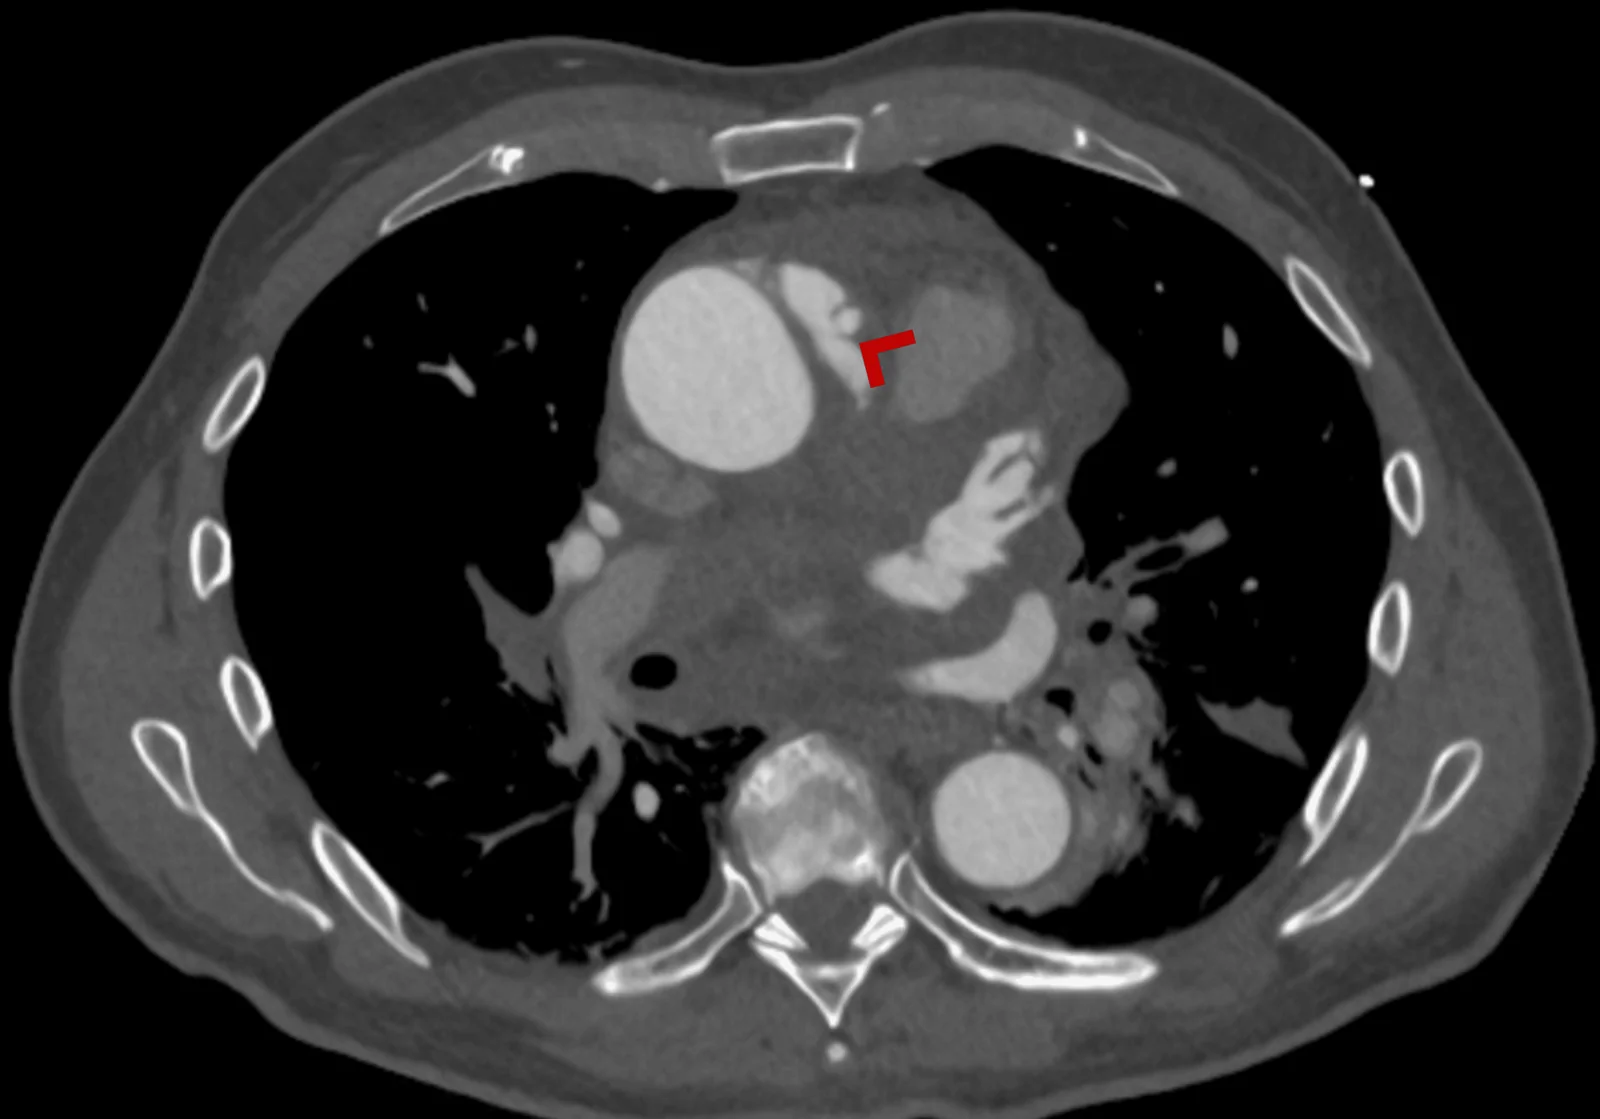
CT scan, axial arterial phase imaging. The red arrow indicates a focal outpouching of the ascending aorta, with surrounding haemopericardium; these features overall are in keeping with shared sheath aortic rupture.
